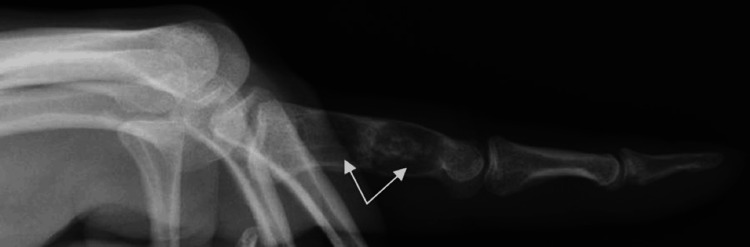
Preoperative X-rays (profile view) indicating the intraosseous lesions in the proximal phalanx of the index finger of the left hand (white arrows).
